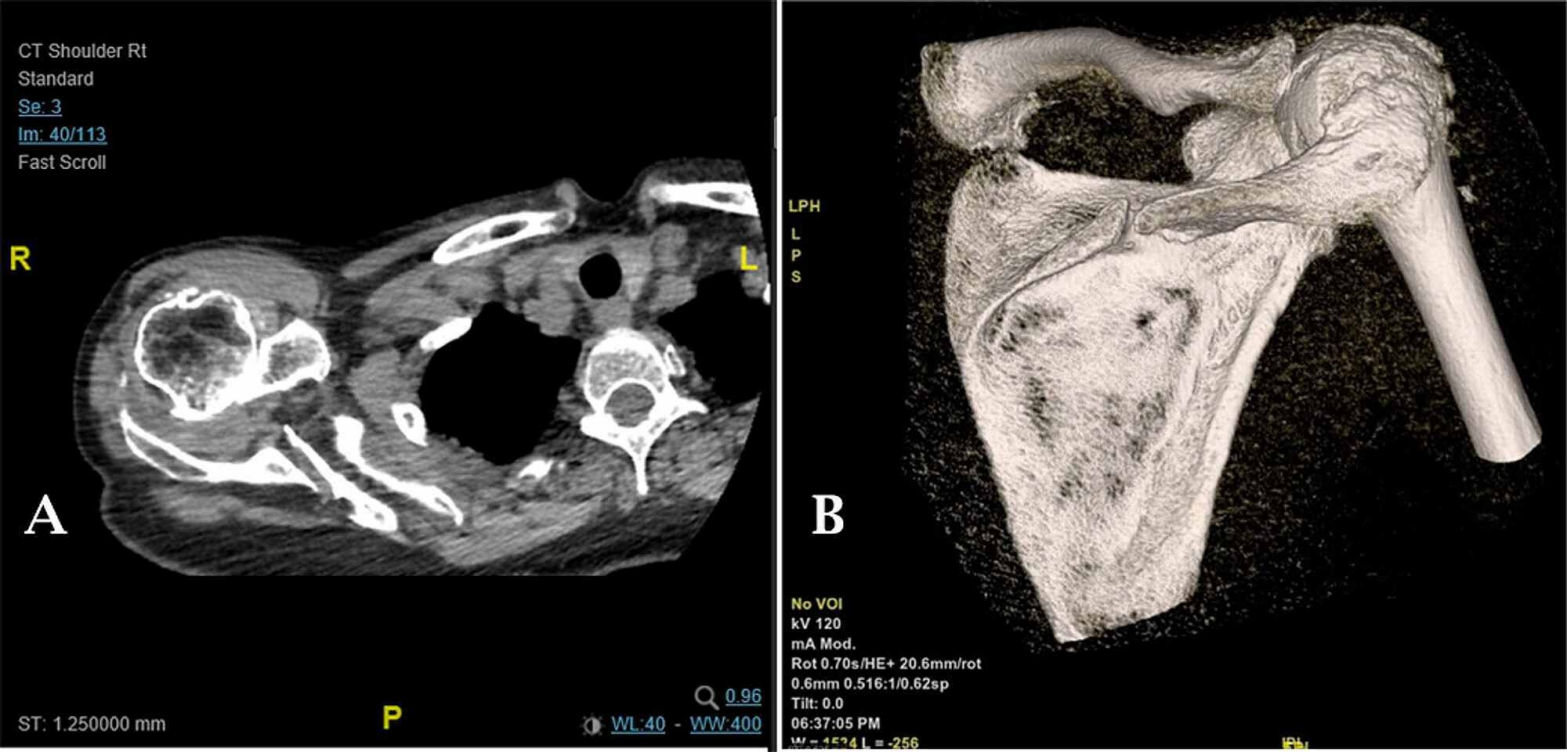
CT images of the right shoulder highlighting a fracture to the spine of the scapula A. Axial CT scan; B. Three-dimensional reconstruction of the CT scan CT: computed tomography
